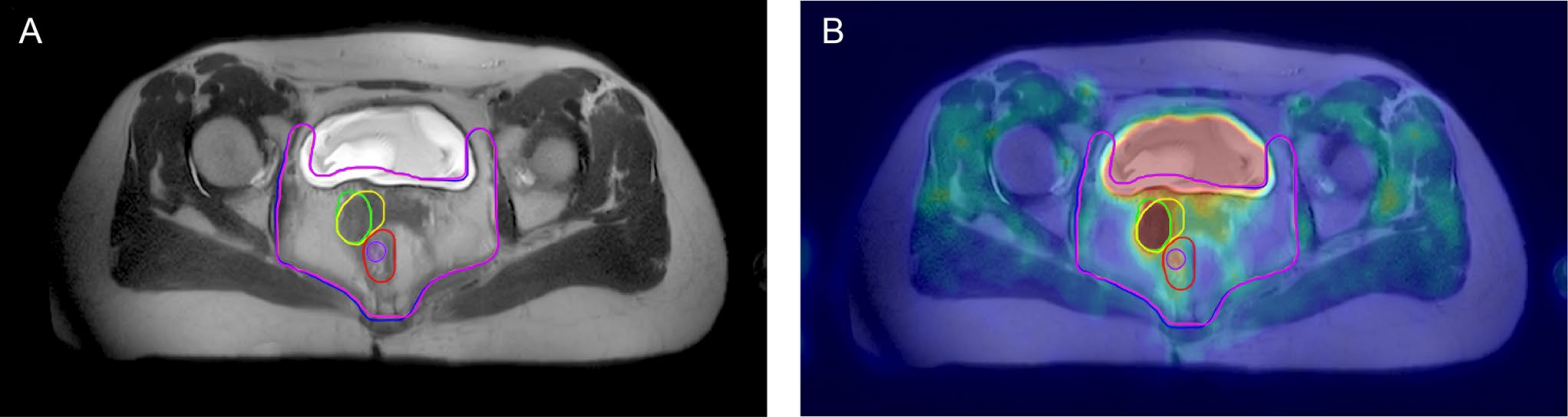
GTV and GTVn delineated for a 38 years old female in red and yellow lines based on MRI (A); GTV and GTVn delineated in purple and green lines based on PET/MRI (B); CTV delineated based on MRI and PET/MRI are represented by pink and blue lines, respectively. Combining with the metabolic information of PET, the boundary of the target volume can be more clearly delineated
